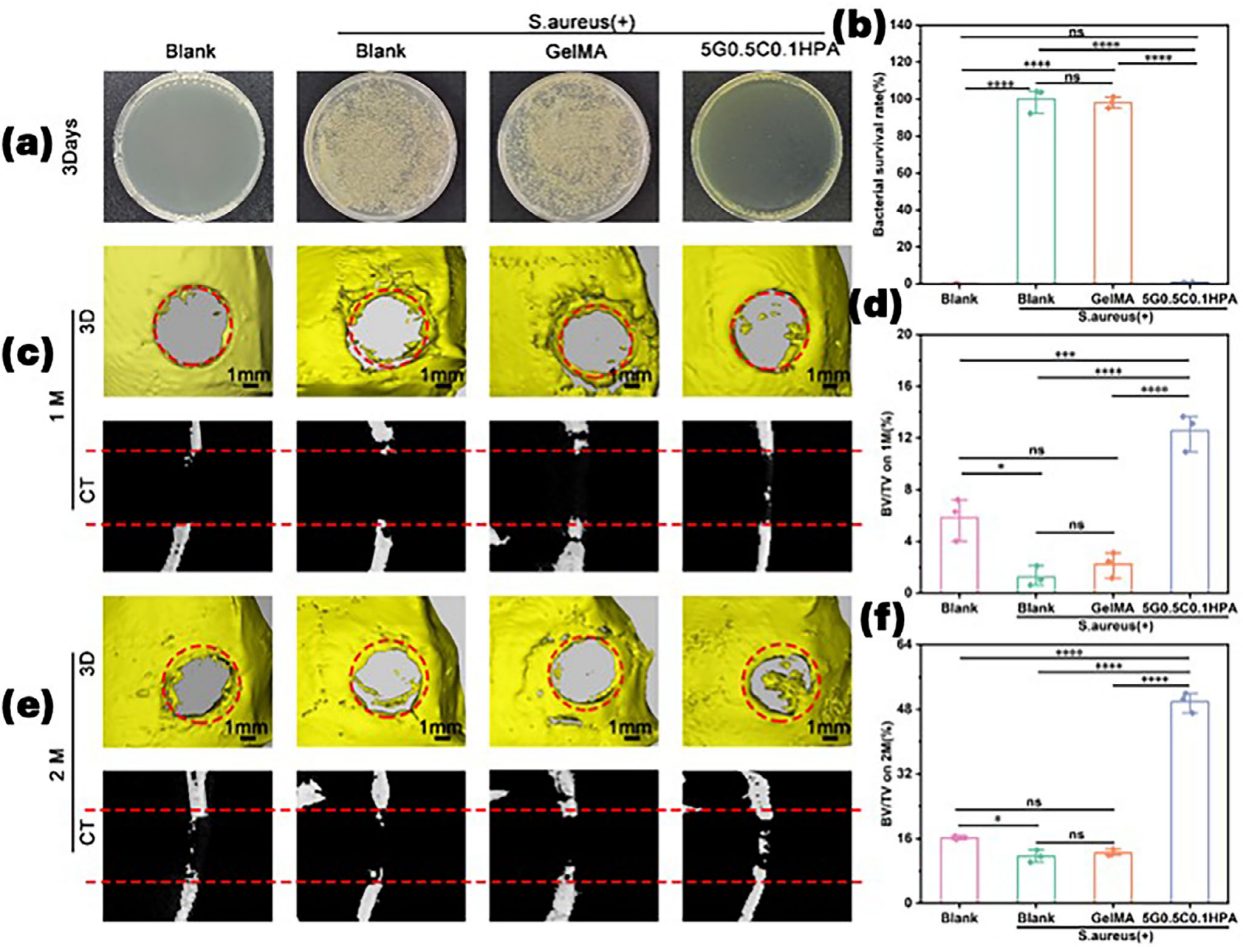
(a) Photographs of in vivo bacteria development after 3 days cultured on the skull defect. (b) Count of the bacterial survival rate cultured from the skull defect. (c) Photographs and CT scan of the bone healing process after 1 month. (d) Measured bone volume fraction after 1 month of treatment. (BV: bone volume; TV: tissue volume). (e) Photographs and CT scan images of the bone healing process after 2 months. (f) Measured bone volume fraction after 1 month of treatment. *p* < 0.05 (*), *p* < 0.01 (), *p* < 0.001 (*), *p* < 0.0001 ().
